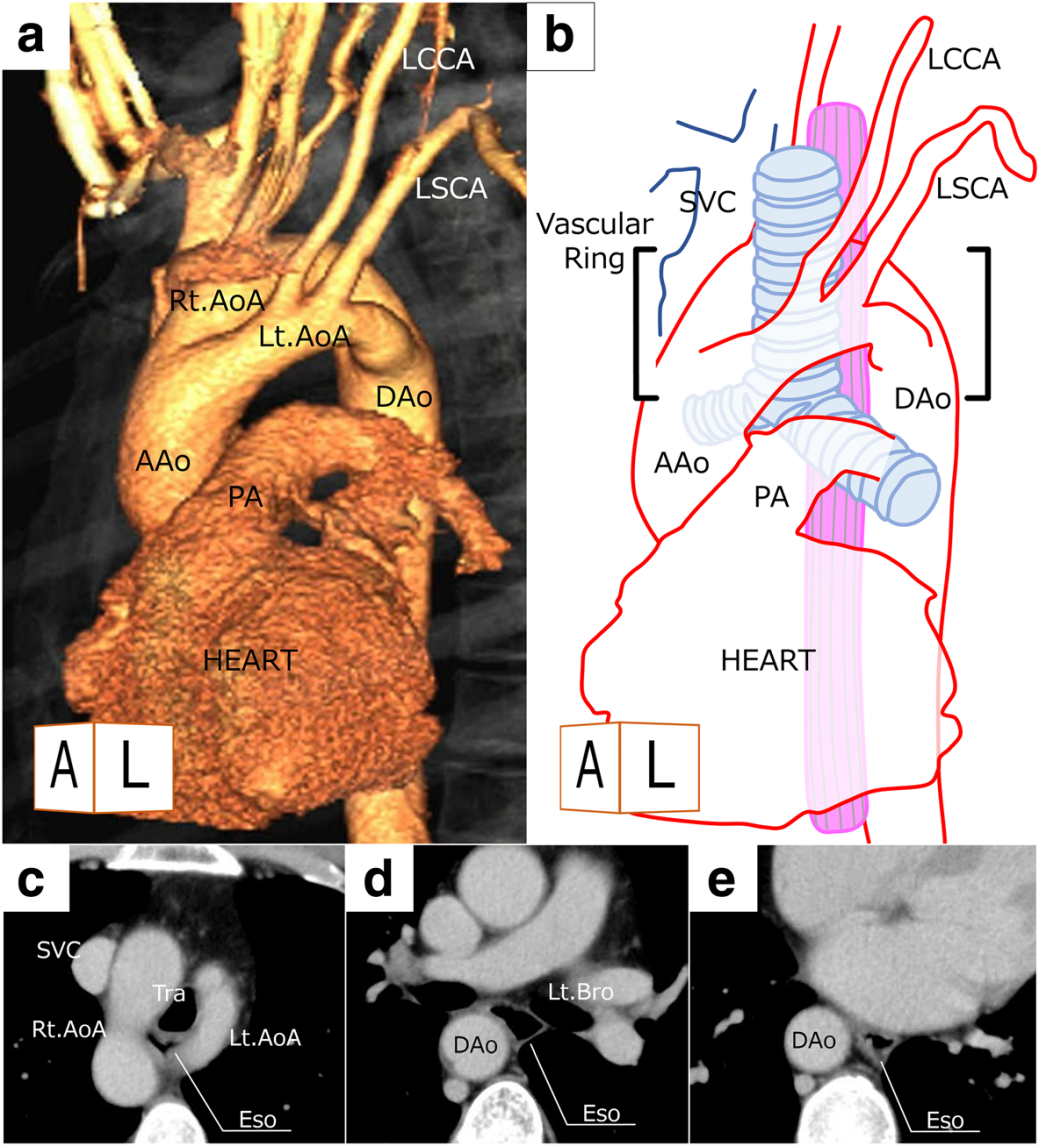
a, b Three-dimensional CT showed a double aortic arch. c–e Axial views on CT revealed the location of the esophagus and descending aorta. Approaching the esophagus from the right side of the thoracic cavity was expected to be difficult. AAo, ascending aorta; DAo, descending aorta; Eso, esophagus; Lt./Rt. AoA, left/right aortic arch; Lt. Bro, left bronchus; LCCA, left common carotid artery; LSCA, left subclavian artery; Tra, trachea
